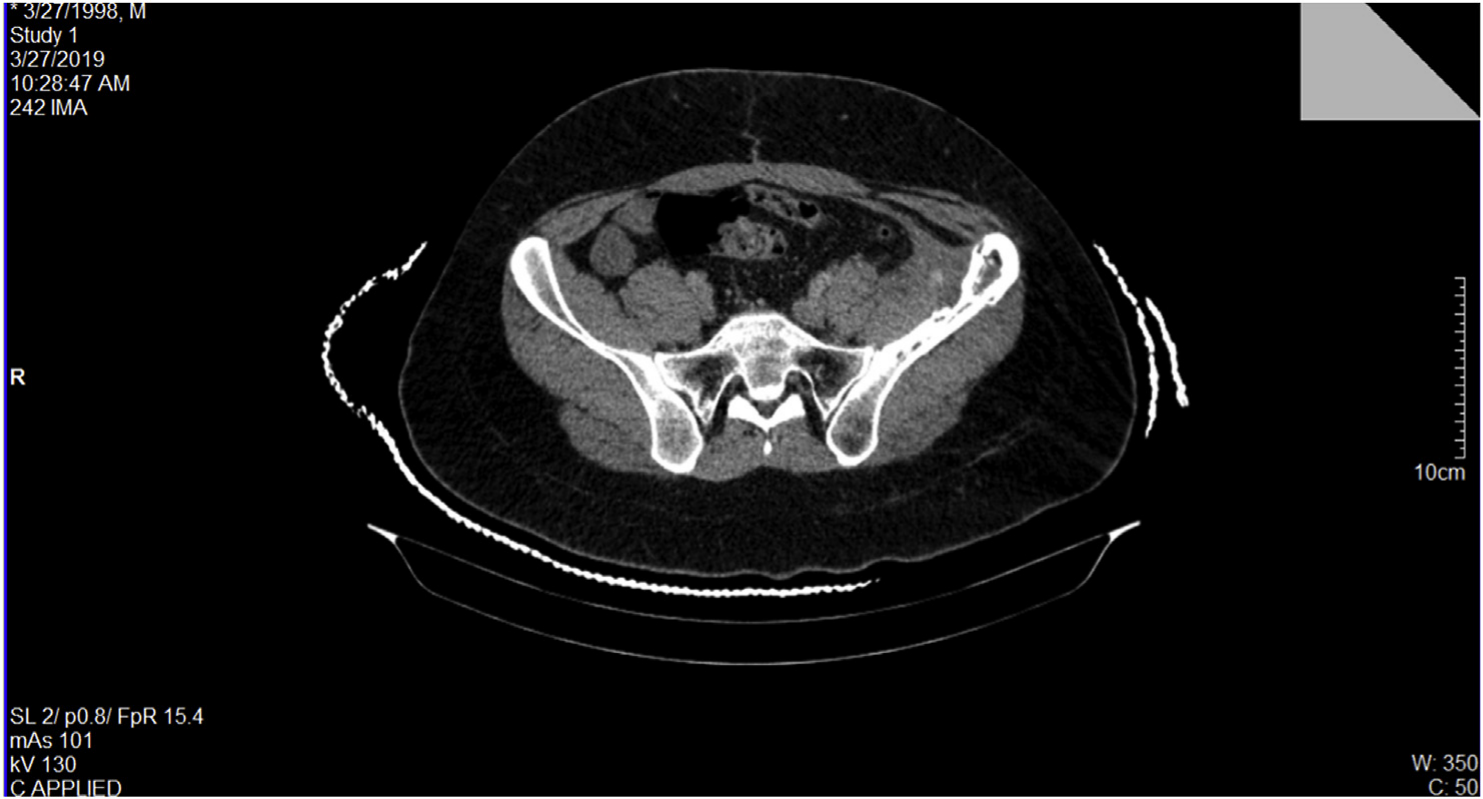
The last follow-up CT scan showing the residual erosion of the iliac crest without any evolvement of the tumor.
